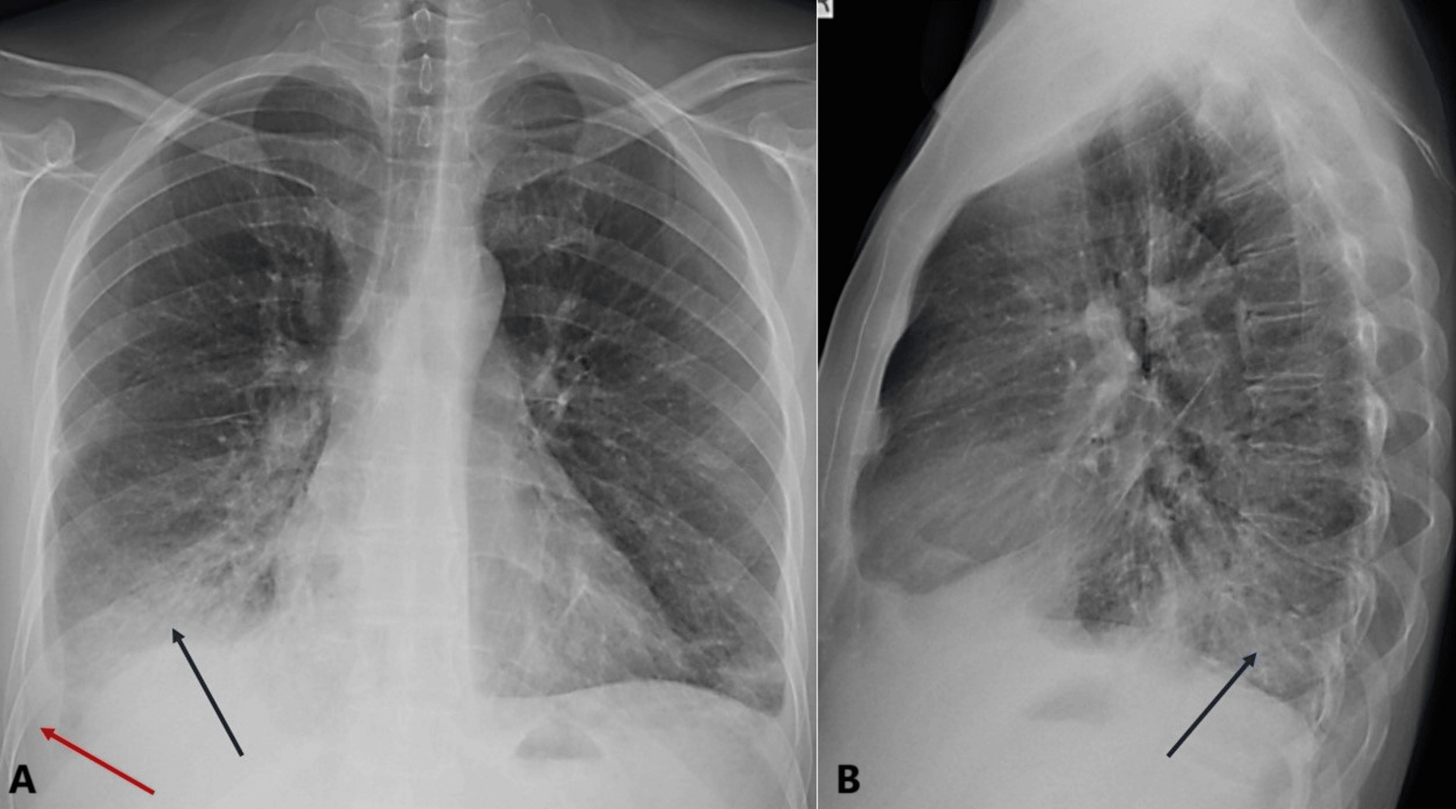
Chest X-ray on day 4 of hospitalization (A) Posterior-anterior view showing worsening right lower lobe infiltrates (blue arrow) and pleural effusion (red arrow), indicating progression of the pneumonia despite initial antibiotic therapy. (B) Lateral view highlighting the same findings (blue arrow), further demonstrating the disease progression.
